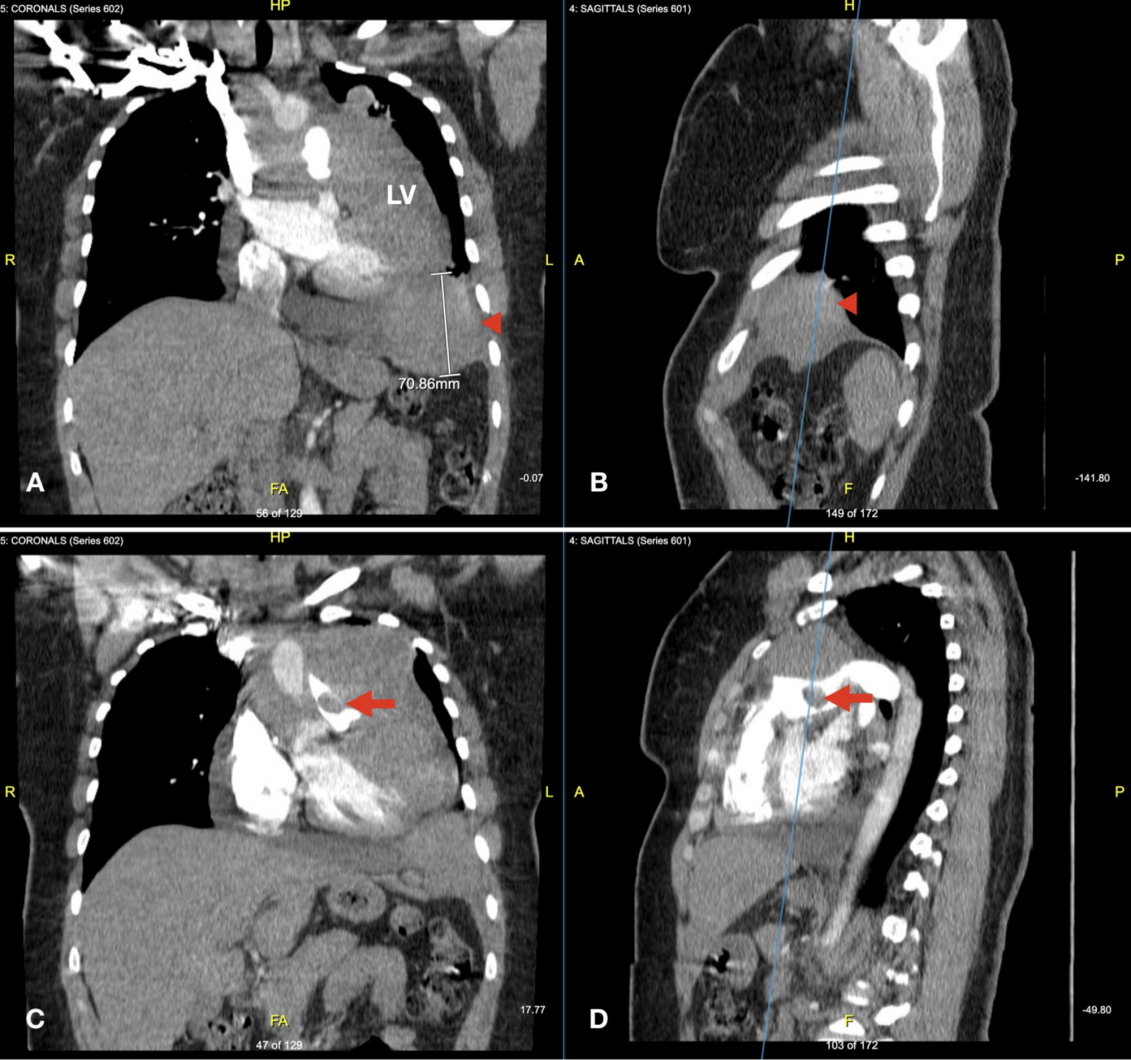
CT angiography coronal and sagittal reconstruction Coronal (A, C) and sagittal (B, D) reconstructions highlight the mass abutting the diaphragm (arrowhead) and infiltrating the left ventricle (LV) free wall (A & B), pulmonary artery involvement, and the intraluminal neoplastic thrombus (arrow) (C & D)
